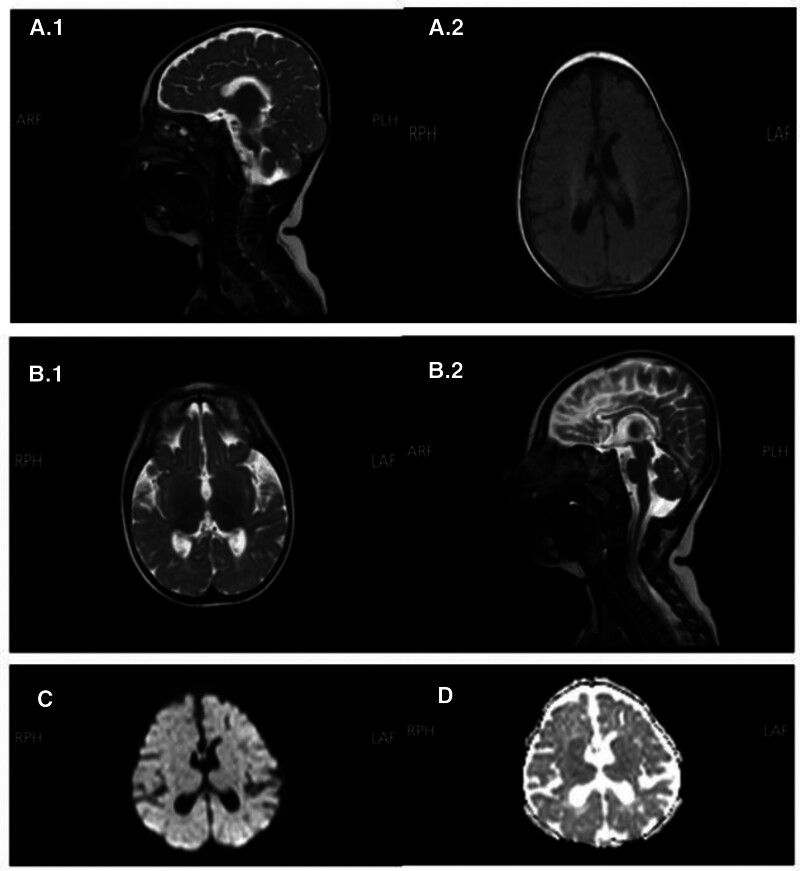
Brain MRI showing a thinning of the corpus callosum, delayed myelination for the patient’s age, and hypoplasia of the anterior horn of the right lateral ventricle. (A.1) T1W MRI sequence in sagittal section, (A.2) T1W MRI sequence in transverse section, (B.1) T2W MRI sequence in sagittal section, (B.2) T2W MRI sequence in transverse section, (C) DWI MRI sequence, and (D) ADC MRI sequence. ADC = apparent diffusion coefficient, MRI = magnetic resonance imaging.
